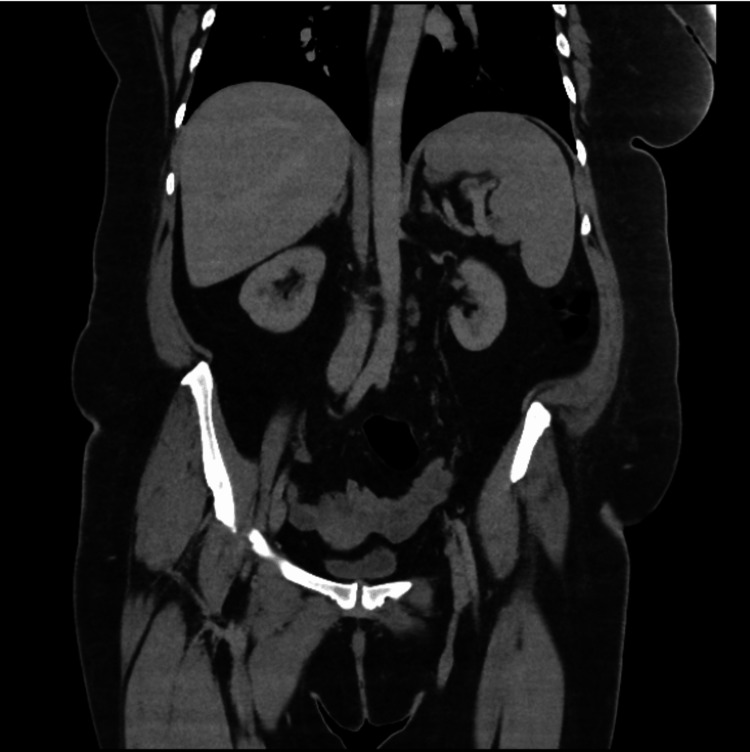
CT abdomen/pelvis (coronal section) revealing no evidence of malignancy
